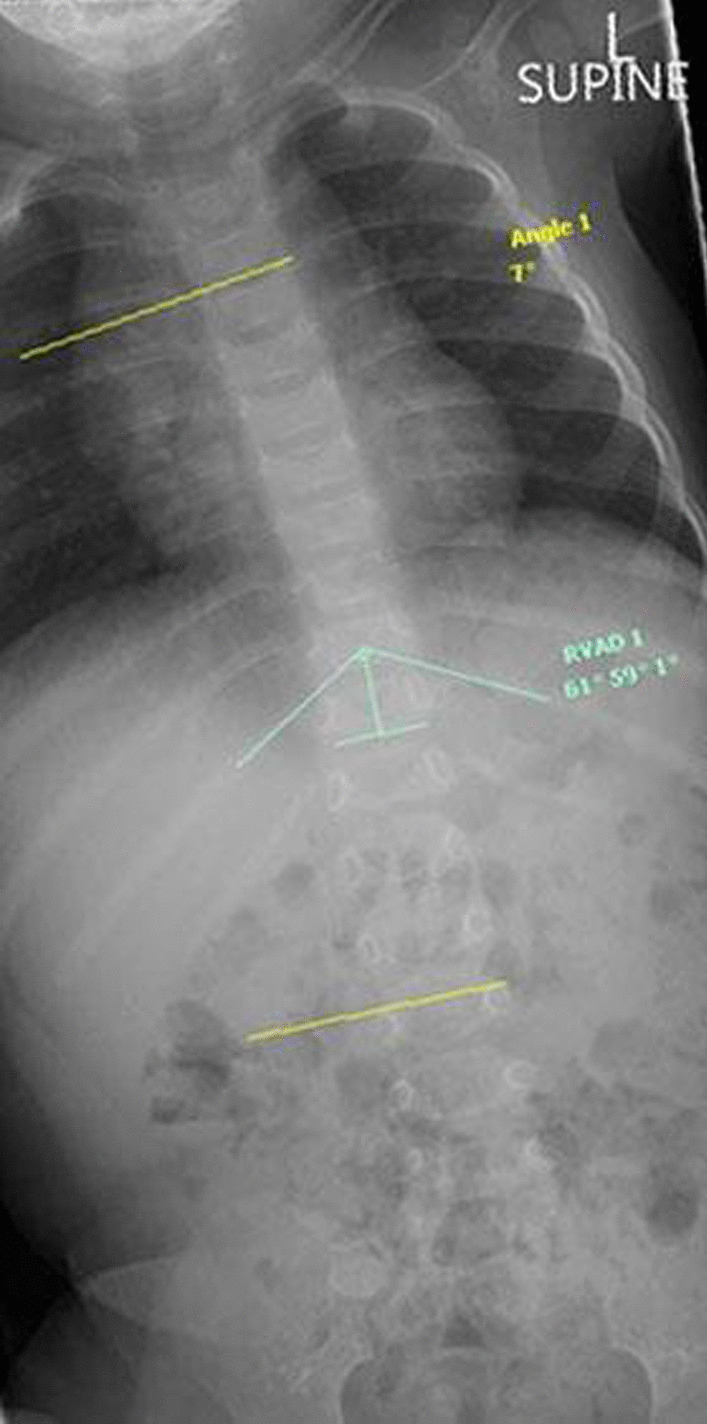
Four months out-of-brace x-ray (supine anteroposterior view). Cobb angle 7°; RVAD 1°
